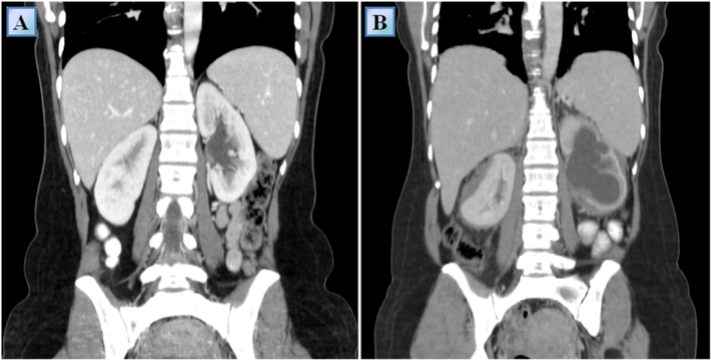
Coronal reformatted contrast enhanced computed tomography images of patient #1, obtained at the time of the diagnosis (A) and 4.5 years after the diagnosis (B). The latter reveals severe hydronephrosis and marked cortical thinning of the left kidney and a peri-renal mass compressing the right kidney. Also, note the fine bilateral peri-renal infiltrate forming a ‘hairy kidney’ appearance.
